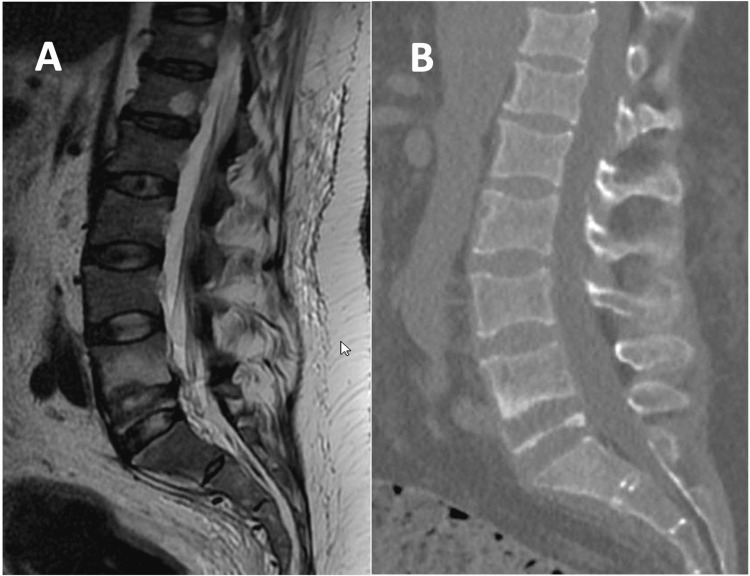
Imaging findings Lumbar spine MRI (A) and CT scan (B) showing complete collapse of the vertebral body of L5. CT, computer tomography; MRI, magnetic resonance imaging
